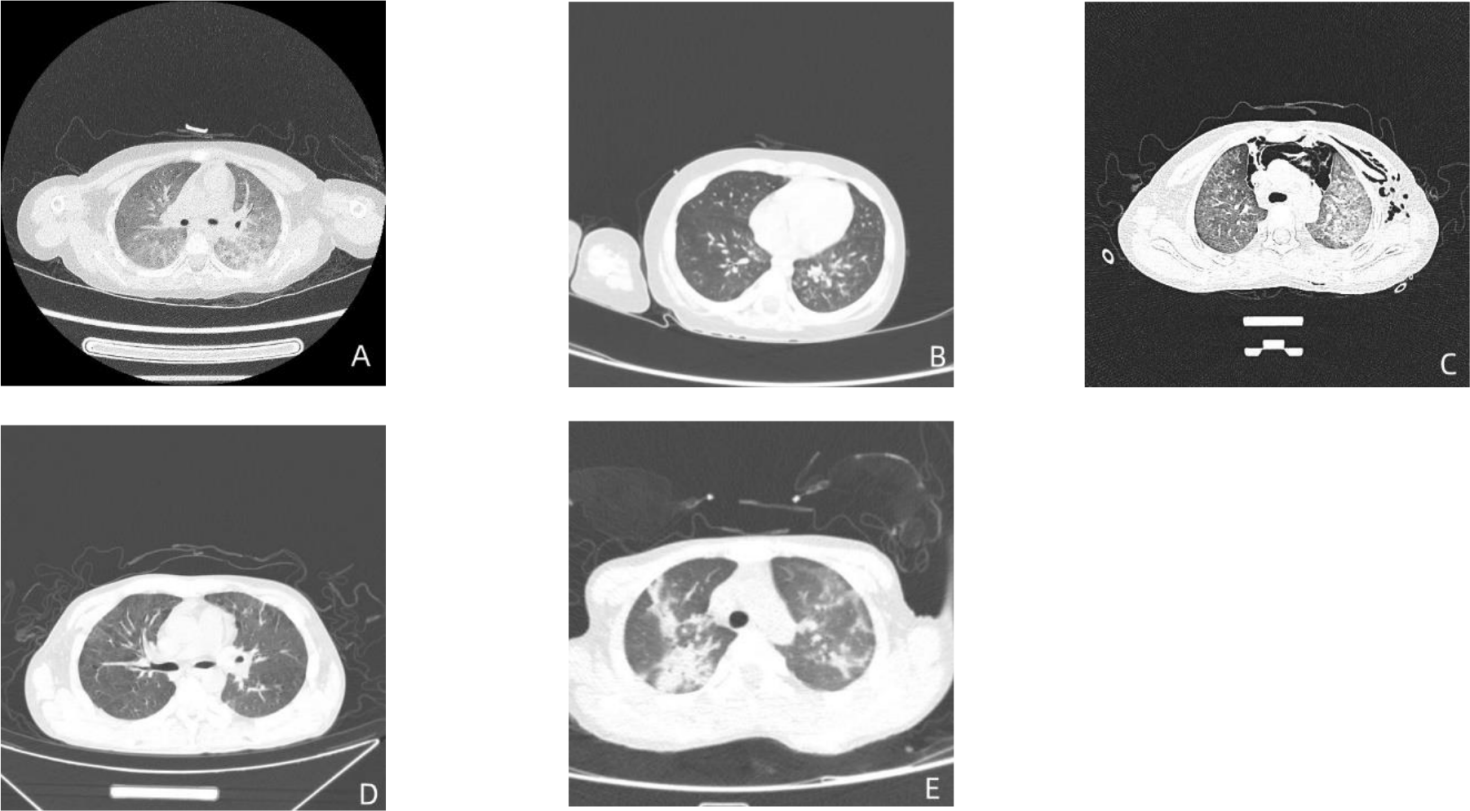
Pulmonary CT results in five children. The figure (A–E) are the CT images of the lungs of case 1-5 in order.
